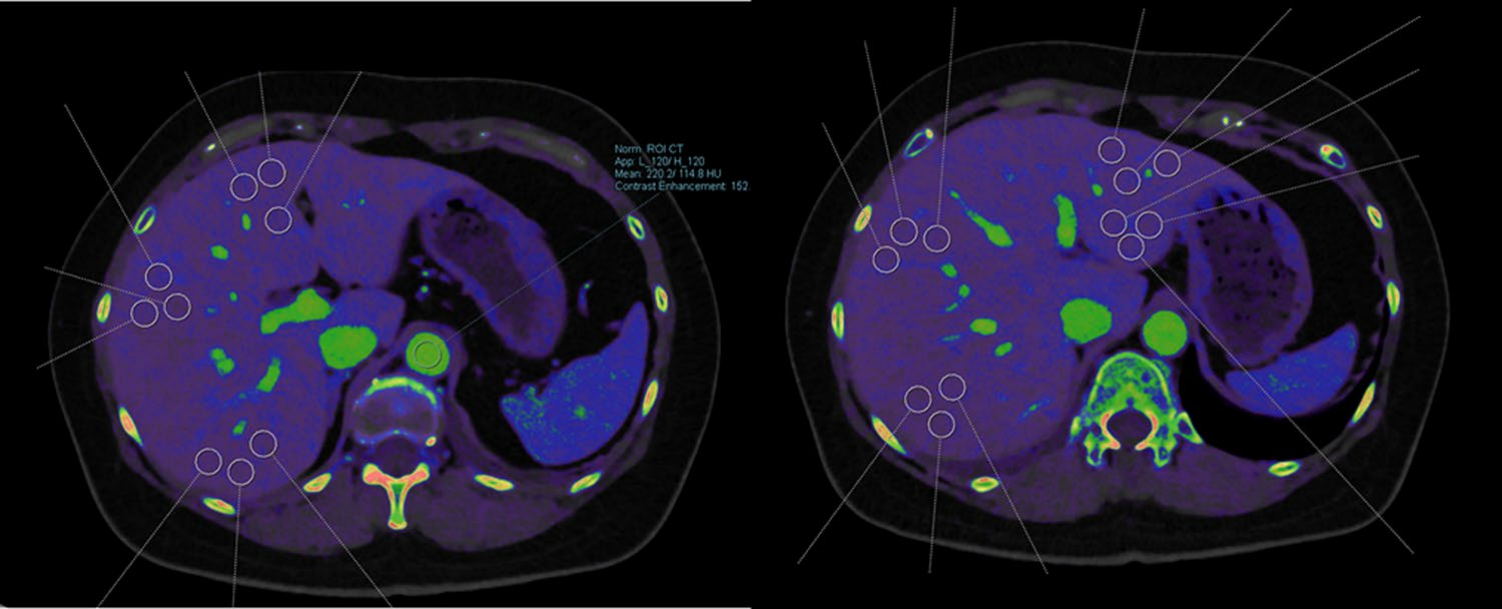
Example of iodine density measurement on an axial PCD-CT image. Iodine density was measured in seven segments (S2–S8) according to Couinaud’s classification. Three 10-mm² circular ROIs were placed within each segment, avoiding focal lesions and large vessels. The average density from these three ROIs represented the segmental iodine density. An ROI was also placed in the aorta
